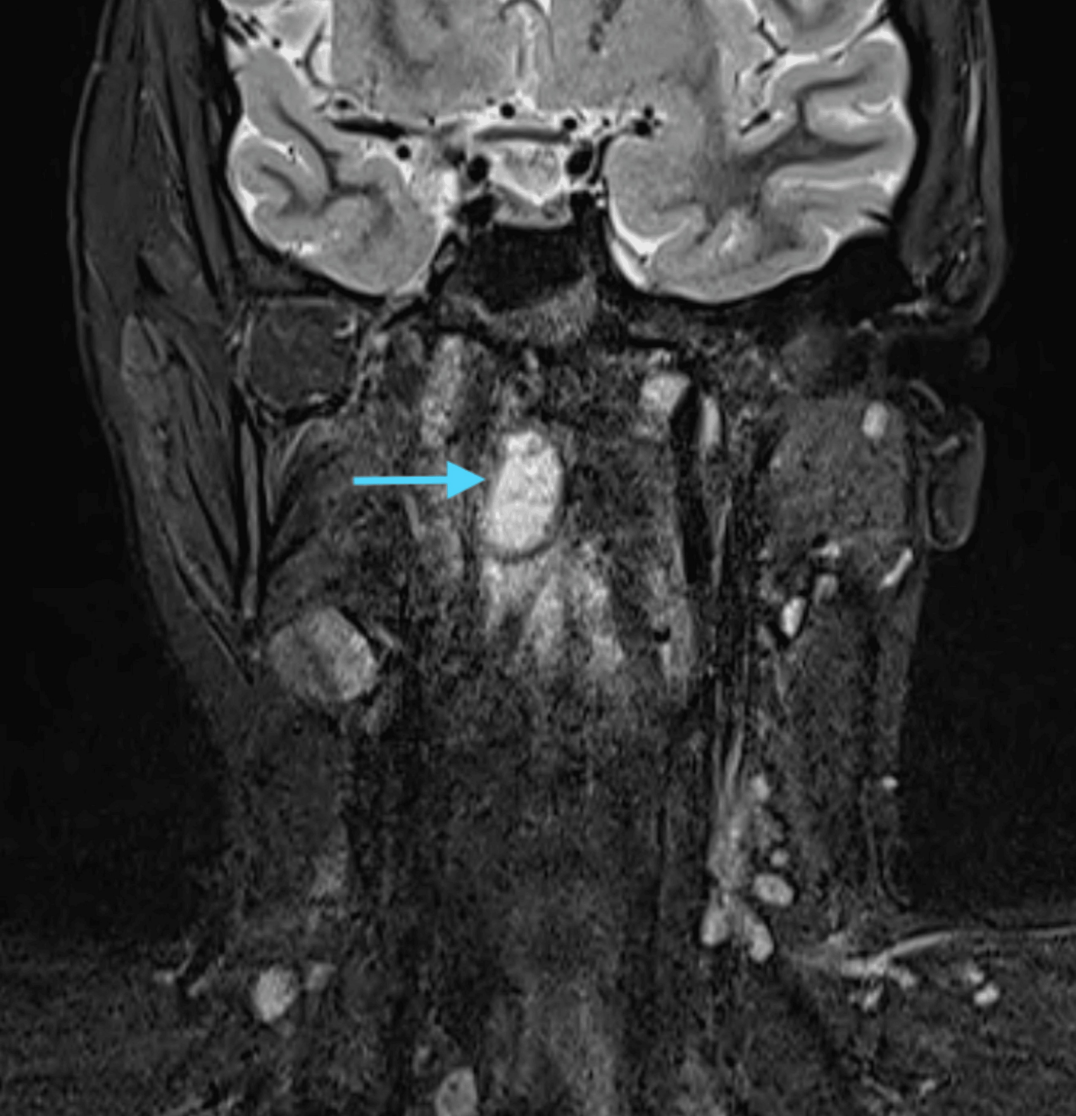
MRI cranio-vertebral junction (contrast) - coronal plane A well-defined lesion measuring approximately 12 x 13 x 26 mm (AP x TR x CC) (blue arrow) is noted in prevertebral soft tissues just on the right side of the midline at the C1-C2 level. It appears hyperintense on STIR and shows peripheral rim enhancement with central non-enhancing hypointense areas suggestive of liquefaction.
